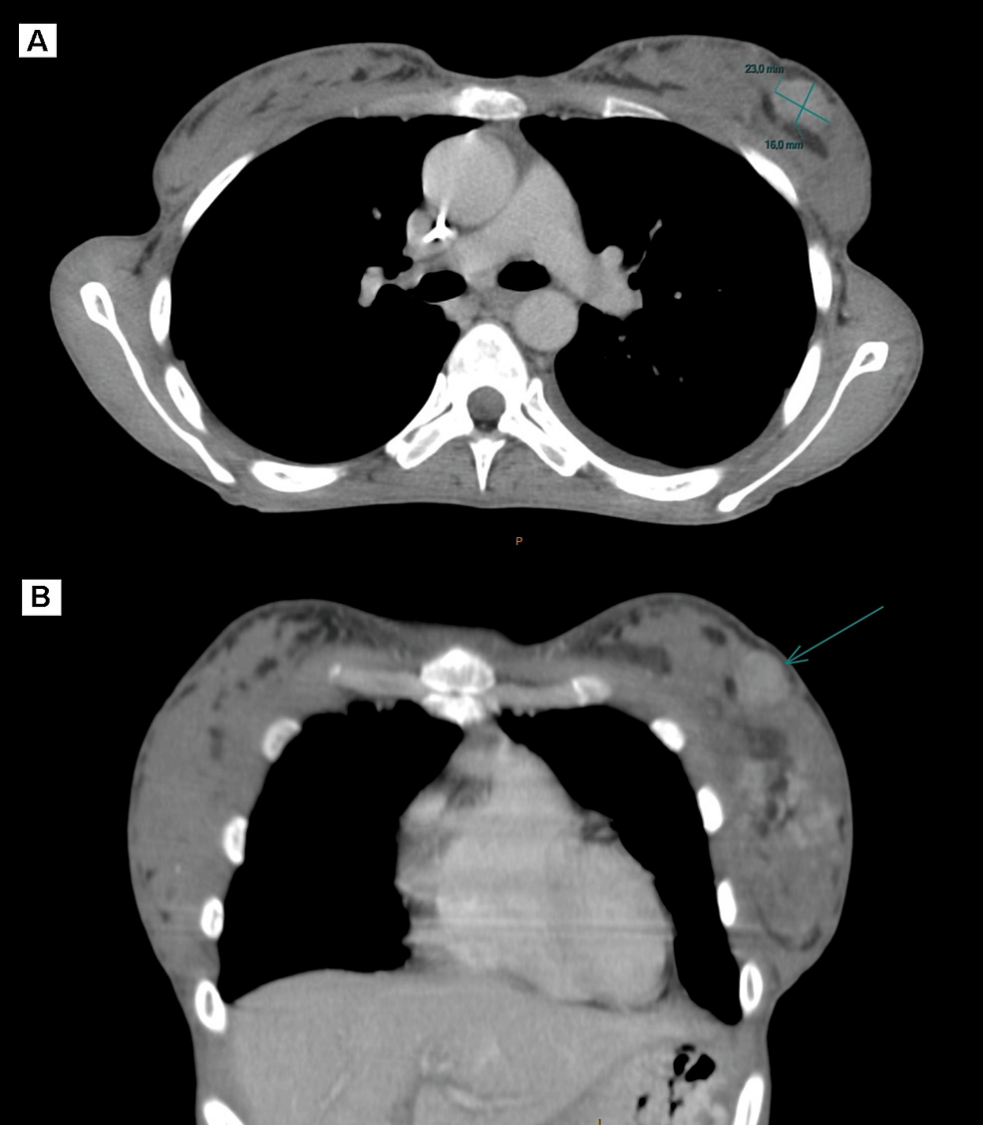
Axial (A) and Coronal (B) CT scan images (arrow) Showing a breast metastasis in the upper outer quadrant of the left breast with a diameter of 26 mm.
